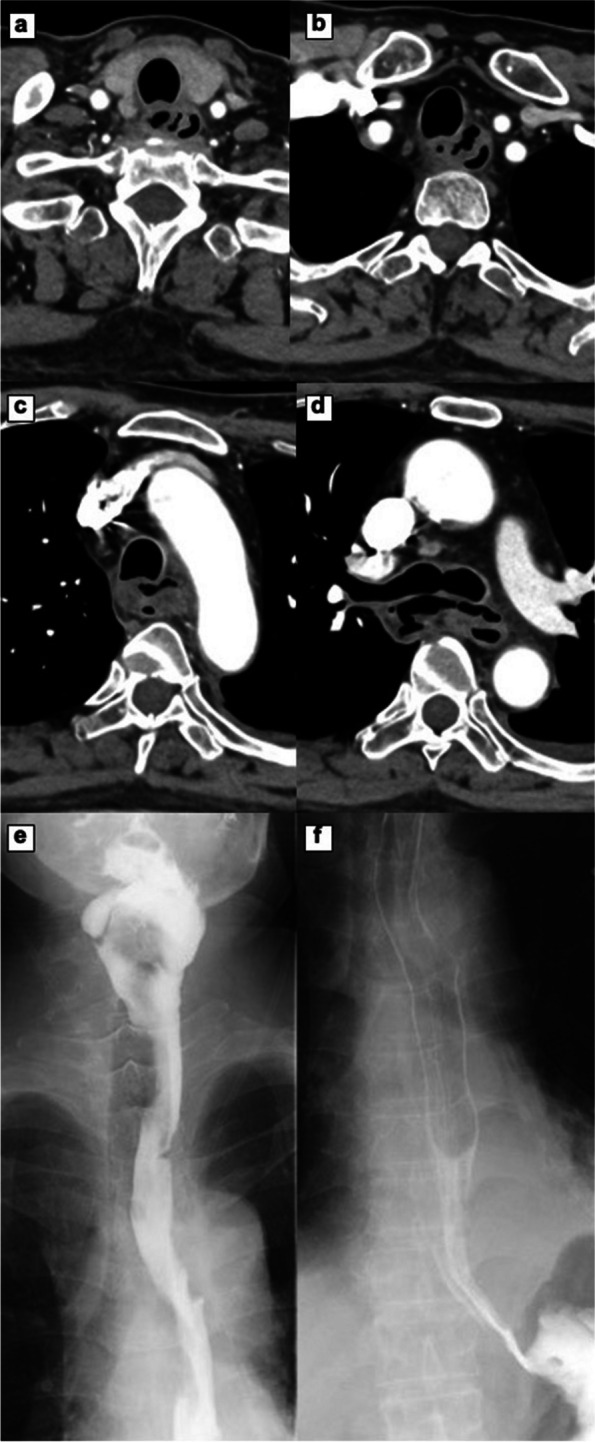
a–d CT revealed mediastinal emphysema and fluid accumulation around the cervical and thoracic esophagus. e, f Upper gastrointestinal radiography using gastrografin showed that there is no leak of the contrast from the esophagus. CT; computed tomography
